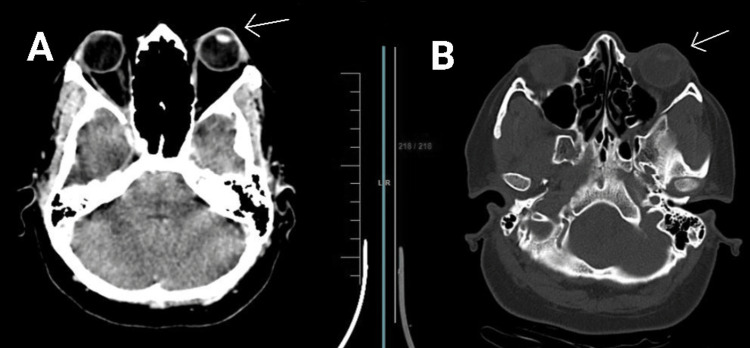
CT brain scan of the patient shows the proptosis of the left eye. (A) Brain window. (B) Bone window. Arrows indicate proptosis.
